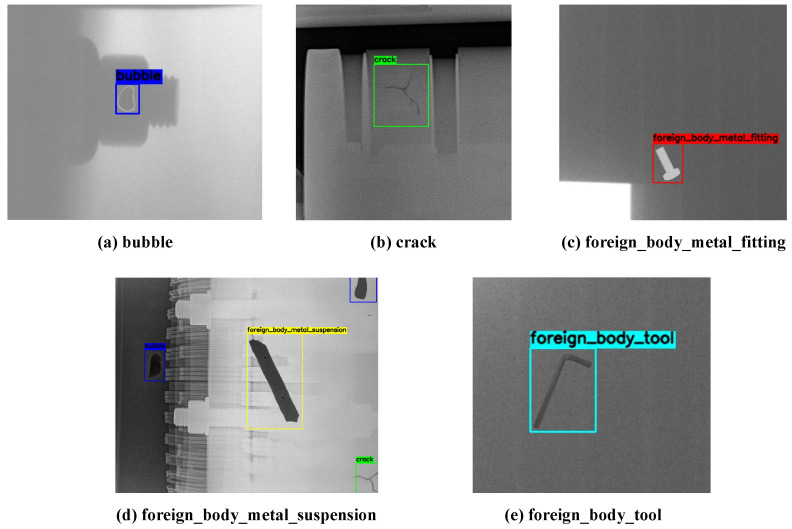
Example images from the custom GIS X-ray dataset, showcasing different defect types discussed in Table 1.
